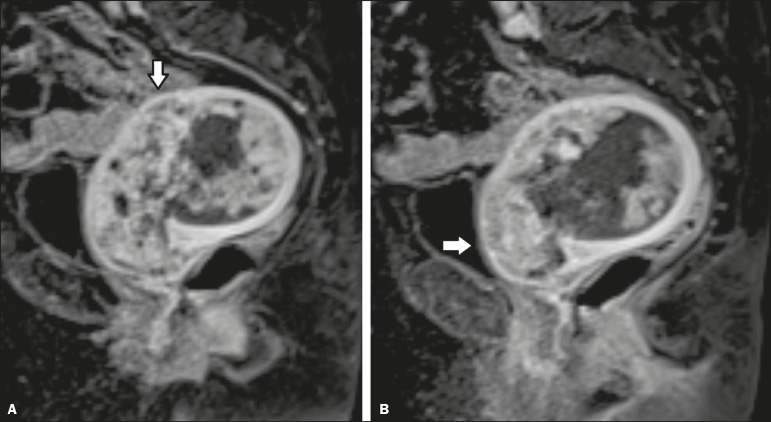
MRI scan from an outside facility showing the pelvis of a 63-year-old
